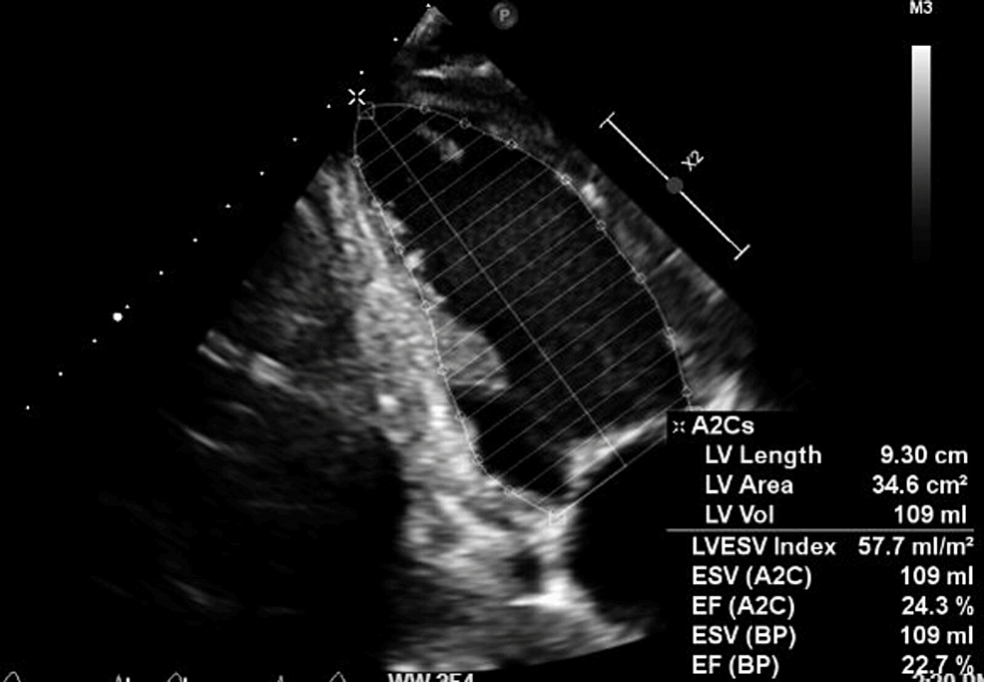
Apical two-chamber view revealing left ventricular measurements in systole and a reduced ejection fraction measurement
